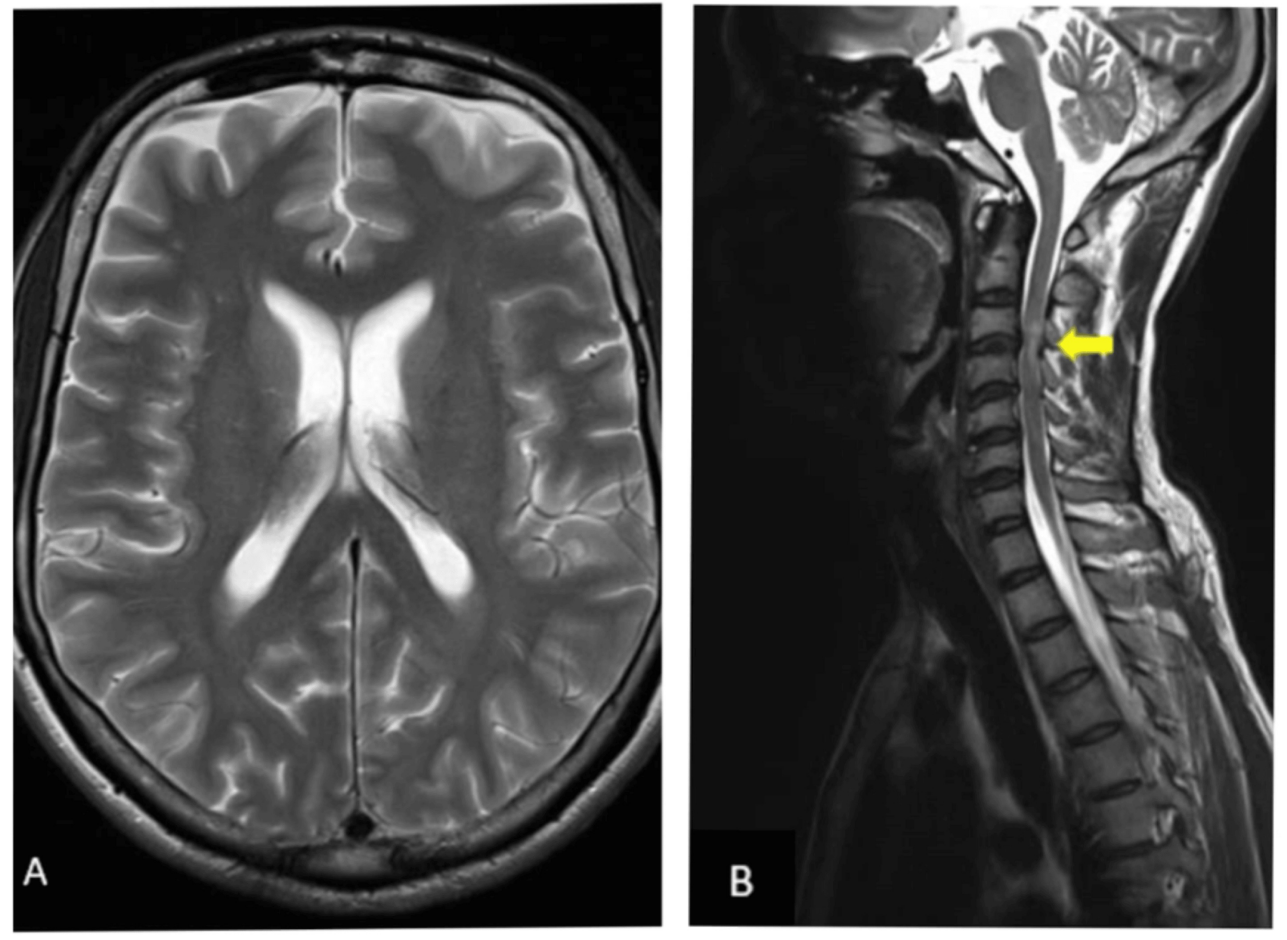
(A) The T2-weighted MRI axial view of the brain showing no abnormality. (B) The T2-weighted MRI sagittal view of the cervical spine showing disc bulge with the altered signal intensity of cord (yellow arrow), suggestive of prolapsed intervertebral disc, a chronic nonspecific finding
